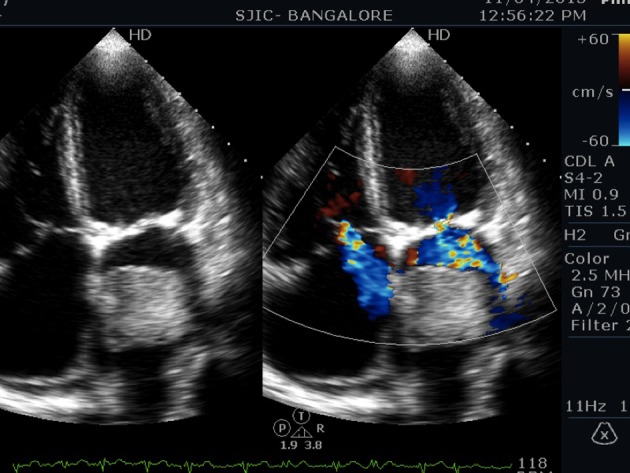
Apical four-chamber view showing moderate tricuspid regurgitation.
